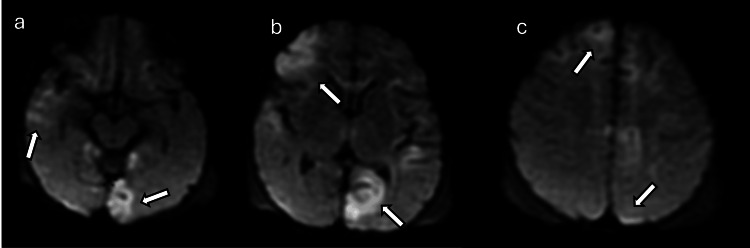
A five-day-old patient with seizures (a), (b), and (c): Axial DWI images show multifocal areas of restricted diffusion throughout the cerebral cortex (arrows) with sparing of the deep gray matter (watershed pattern). Images obtained by the authors from the institutional PACS. All images are de-identified and used in accordance with institutional policy. DWI: diffusion-weighted imaging; PACS: picture archiving and communication system
